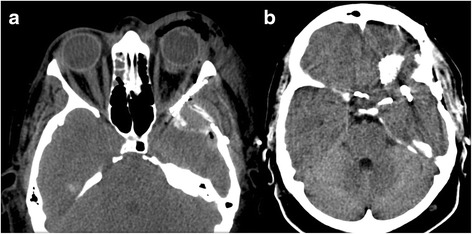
Postoperative computed tomography scans after removal of the tumor and clipping of the three aneurysmatic dilatations in a single stage (a, b)
